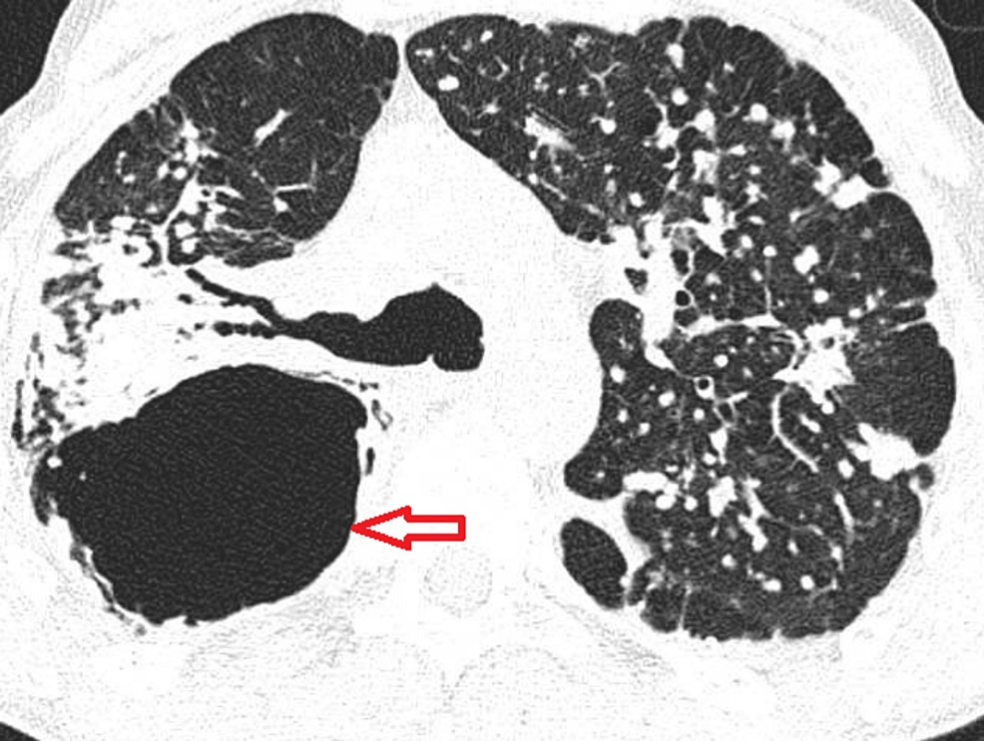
Tuberculosis cavitation observed on chest CT (marked with red arrow)
